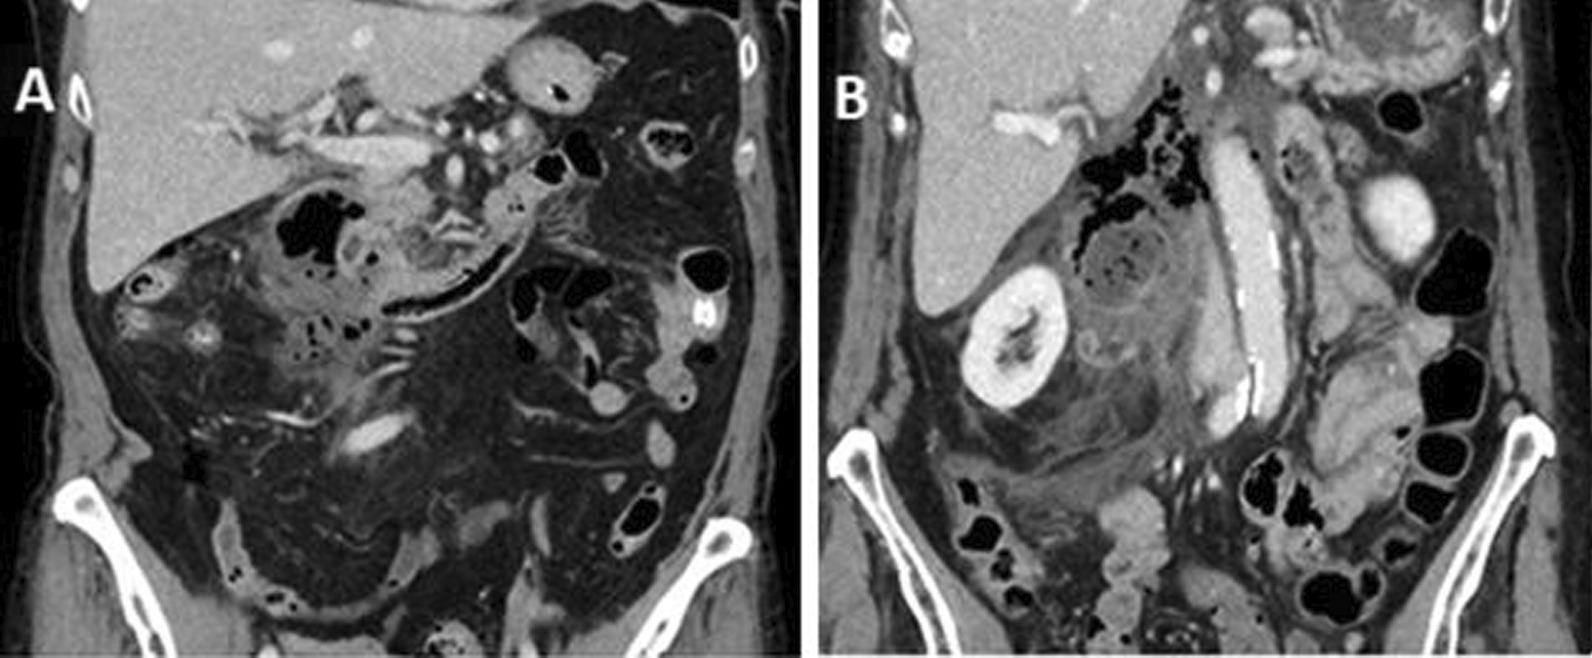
Primary CT-scan of Patient #1 (a) and Patient #2 (b)
